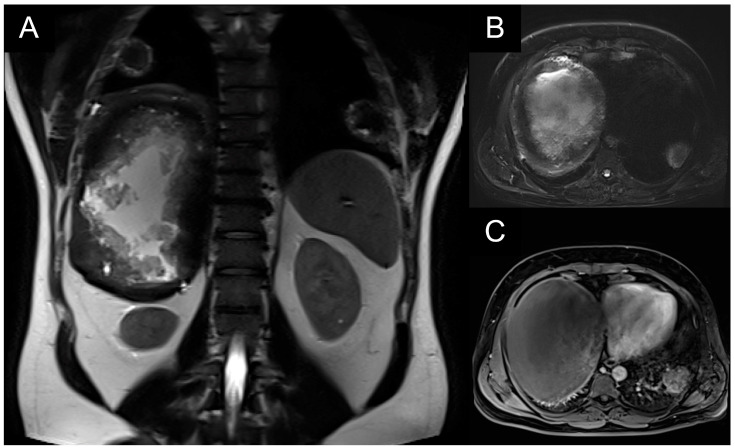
(A,B): T2-weighted hyperintense liver lesion; (C): T1-weighted hypointense liver lesion with abnormal pulmonary lesions.
